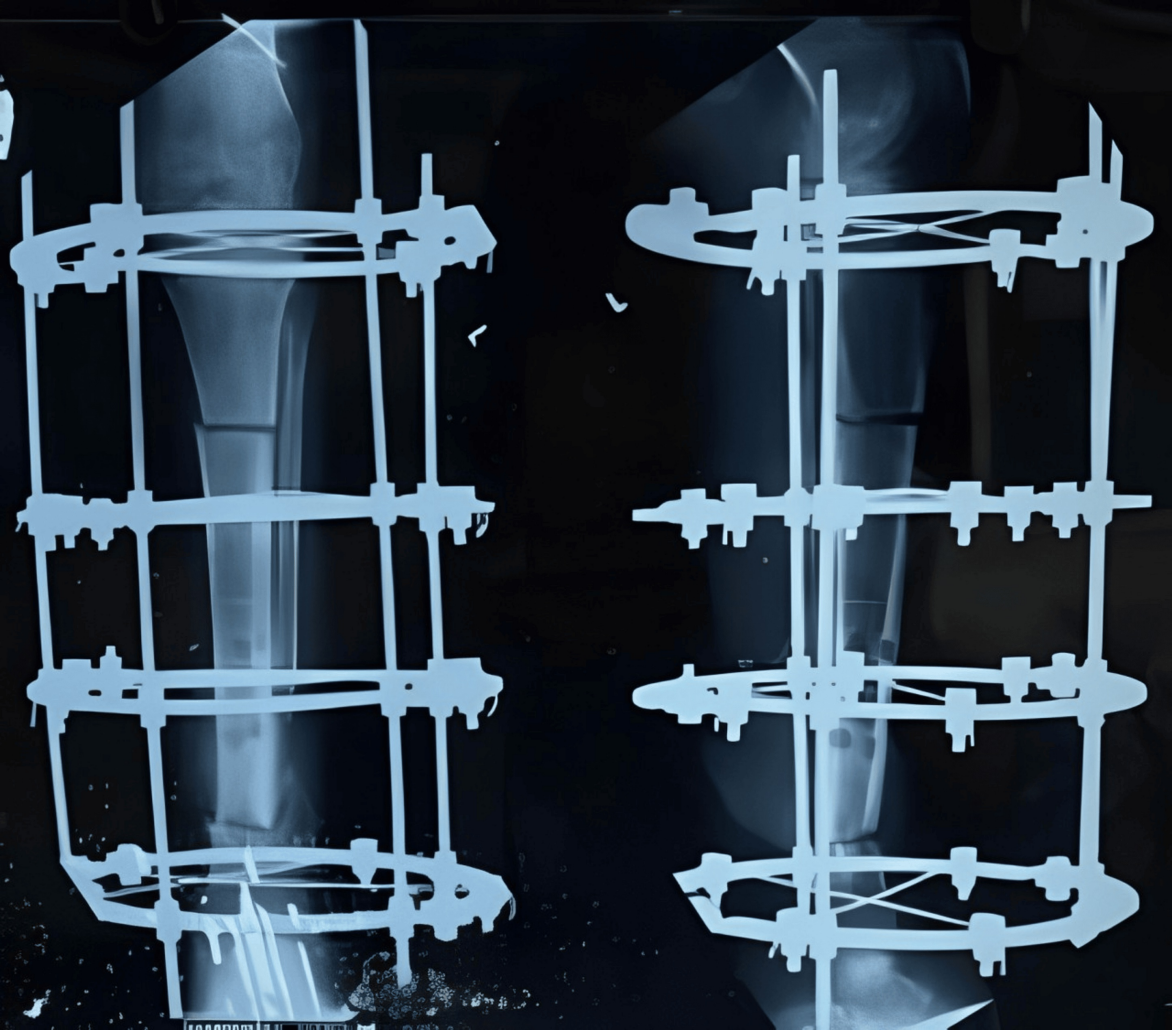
X-ray showing the Ilizarov ring fixator with corticotomy at the proximal site and docking at the distal nonunion site
